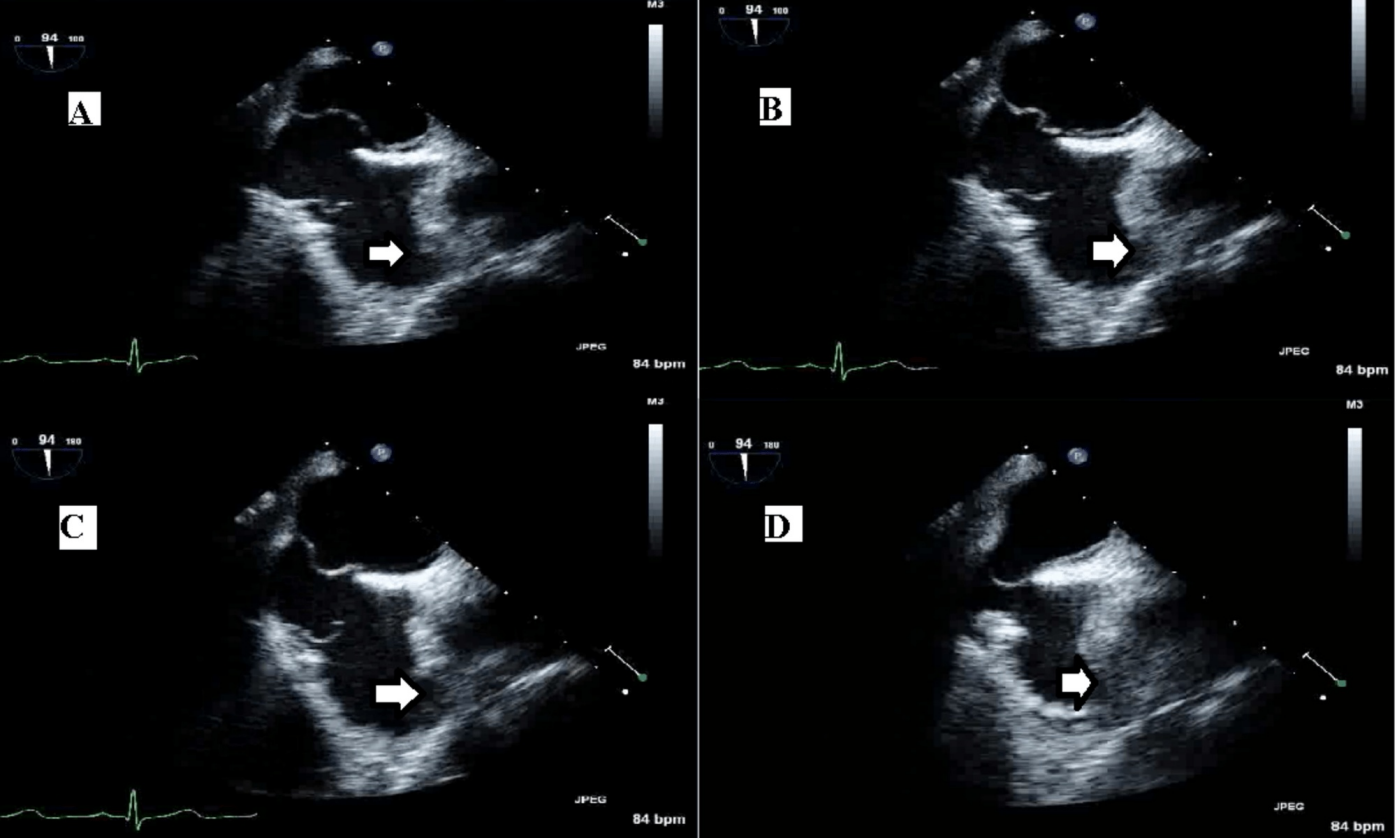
Serial images (A, B, C, D) through transesophageal echocardiogram showing PFO (arrows) and atrial septal aneurysm PFO, patent foramen ovale.
